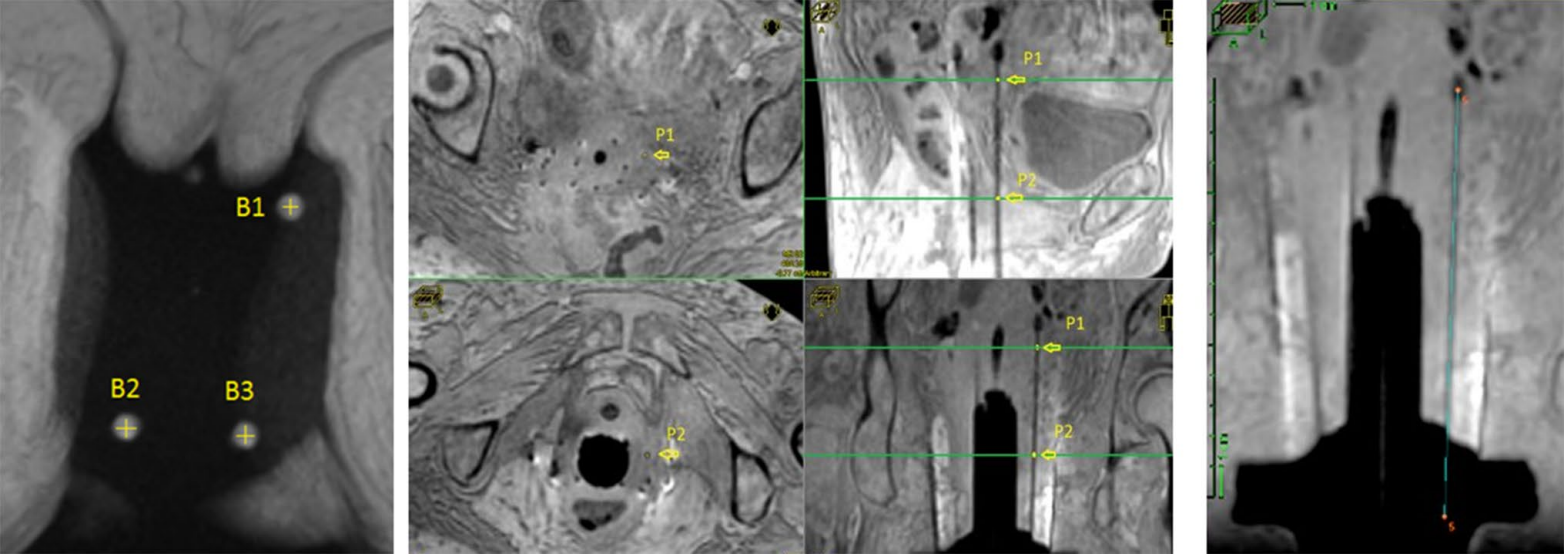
Reconstruction method for titanium needles based on MRI images with the aid of MRI markers (A-vitamin pellets)
